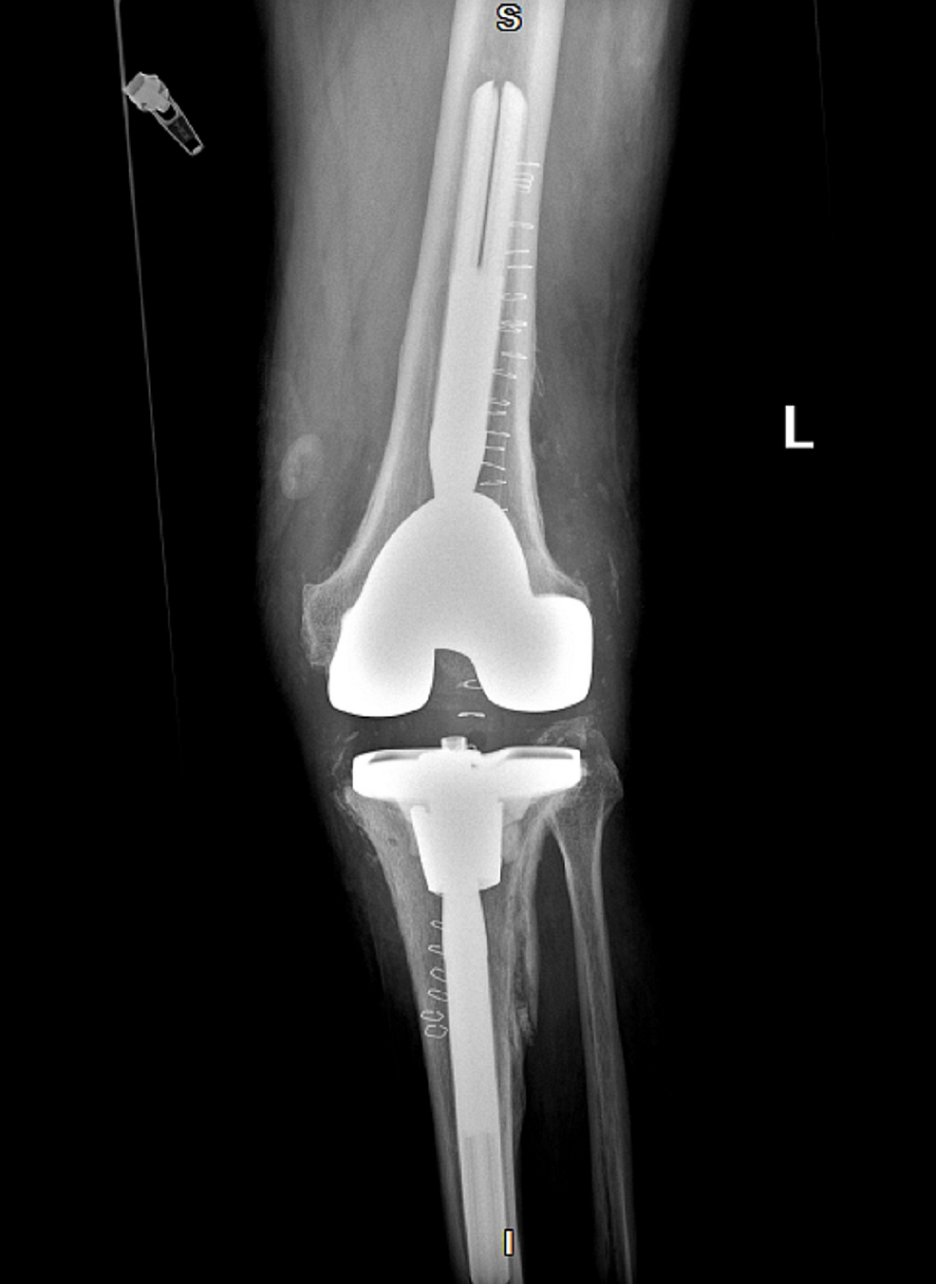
X-ray of the left knee post-antibiotic spacer removal and reimplantation of permanent total knee replacement following the resolution of Abiotrophia infection (anteroposterior)
